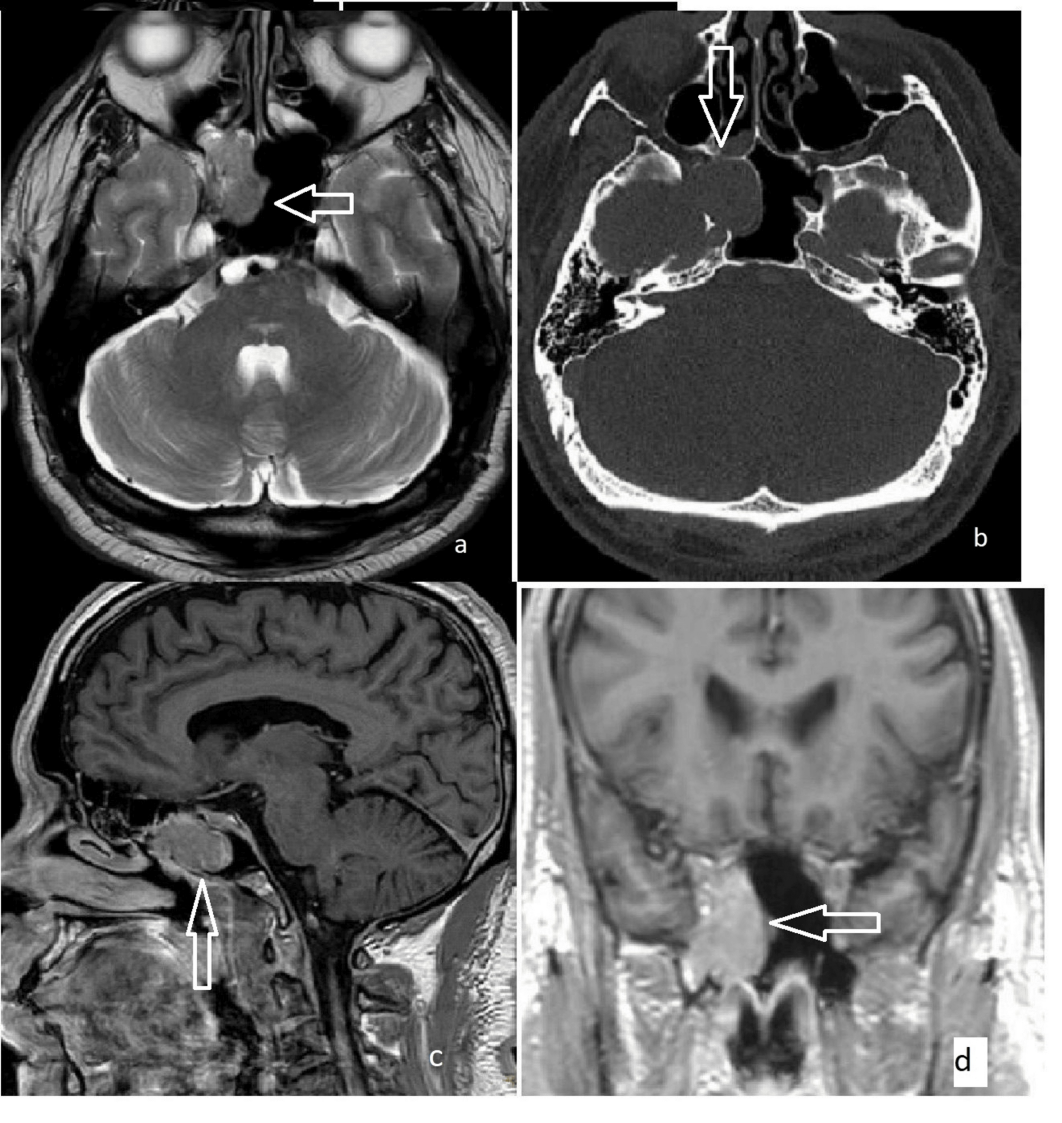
MRI images of the patient showing a hamartoma of the isolated sphenoid sinus (a) axial, (b) CT axial view, (c) sagittal, (d) coronal views.
